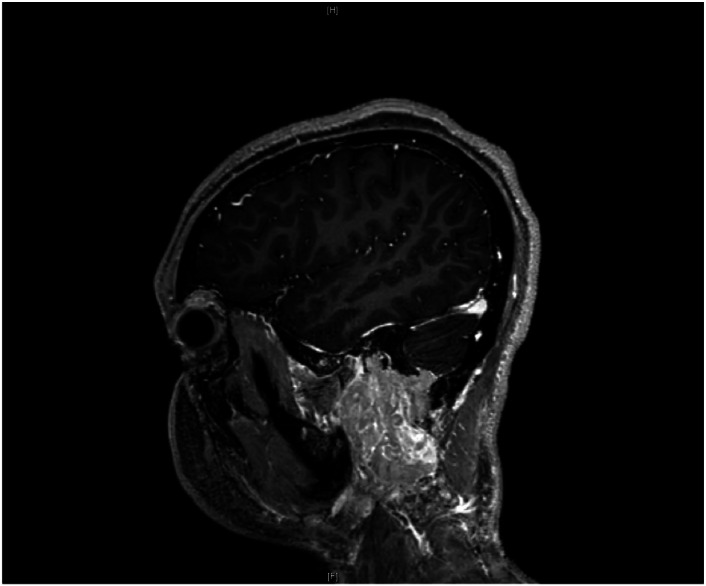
Sagittal MRI demonstrating the large invasive Giant Cell Tumor (GCT). The mass is noted to be extending through the middle ear, into the skull base, and displaces the parotid gland.
